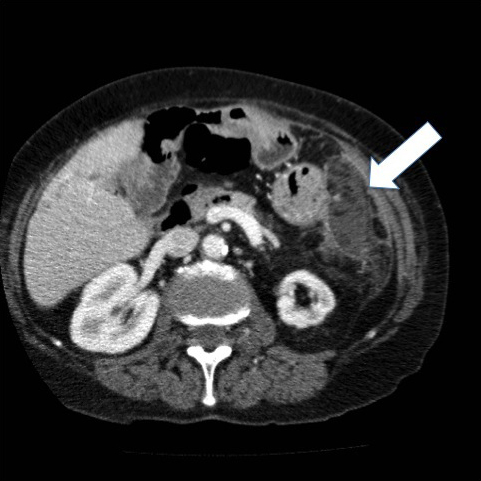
Axial portal venous phase contrast-enhanced CT: arrow shows left upper quadrant fat density mass with peripheral enhancement adjacent to the greater curve of the stomach.
